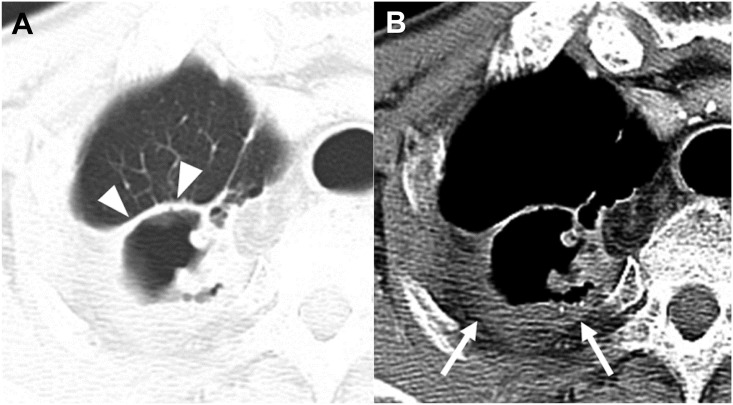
CT images of a *Mycobacterium intracellulare* pulmonary infection in a 77-year-old man. Chest CT axial images were obtained at the level of the right bracheocephalic vein (2.5- mm slice). The lung window setting shows the thin and even thickness of the cavity (arrowheads) in the right upper lobe (A). The mediastinal window setting shows thick pleural thickening with proliferation of extra-pleural fat next to the cavity in the right upper lobe (arrows) (B).
